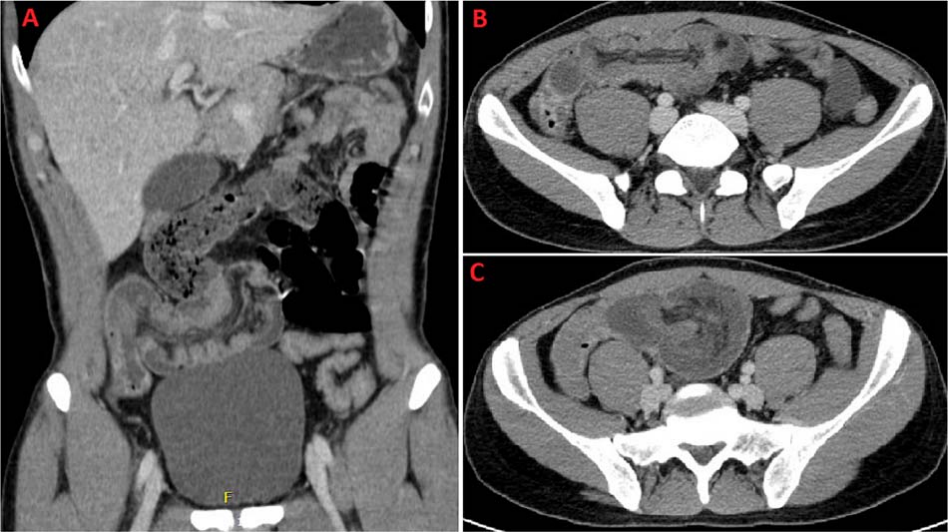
A and B: Coronal and axial sections of contrast-enhanced CT scan confirming wall thickening of the last ileal loop and the cecum up to 20 mm. C: CT imaging performed the following day, displaying increased wall thickening up to 24 mm with the last ileal loop appearing twisted around its mesentery.
